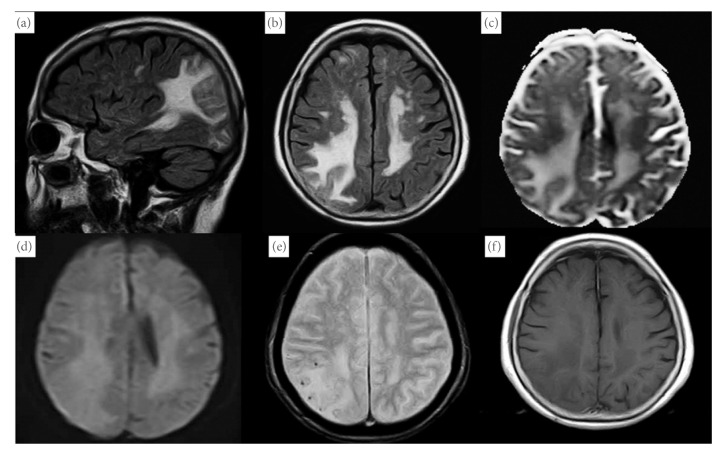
Magnetic resonance imaging (MRI) shows hypersignal intense lesions in the subcortical white matter in the bilateral and asymmetric parietal lobe in both fluid-attenuated inversion recovery (FLAIR) sequence (a and b) and apparent diffusion coefficient (ADC; (c)); however, these lesions were not recognized in diffusion-weighted imaging (DWI; (d)). T2*∗*-weighted gradient echo imaging reveals some subcortical microbleeds in the right parietal lobe (e). Postgadolinium T1-weighted images show no enhancement (f).
